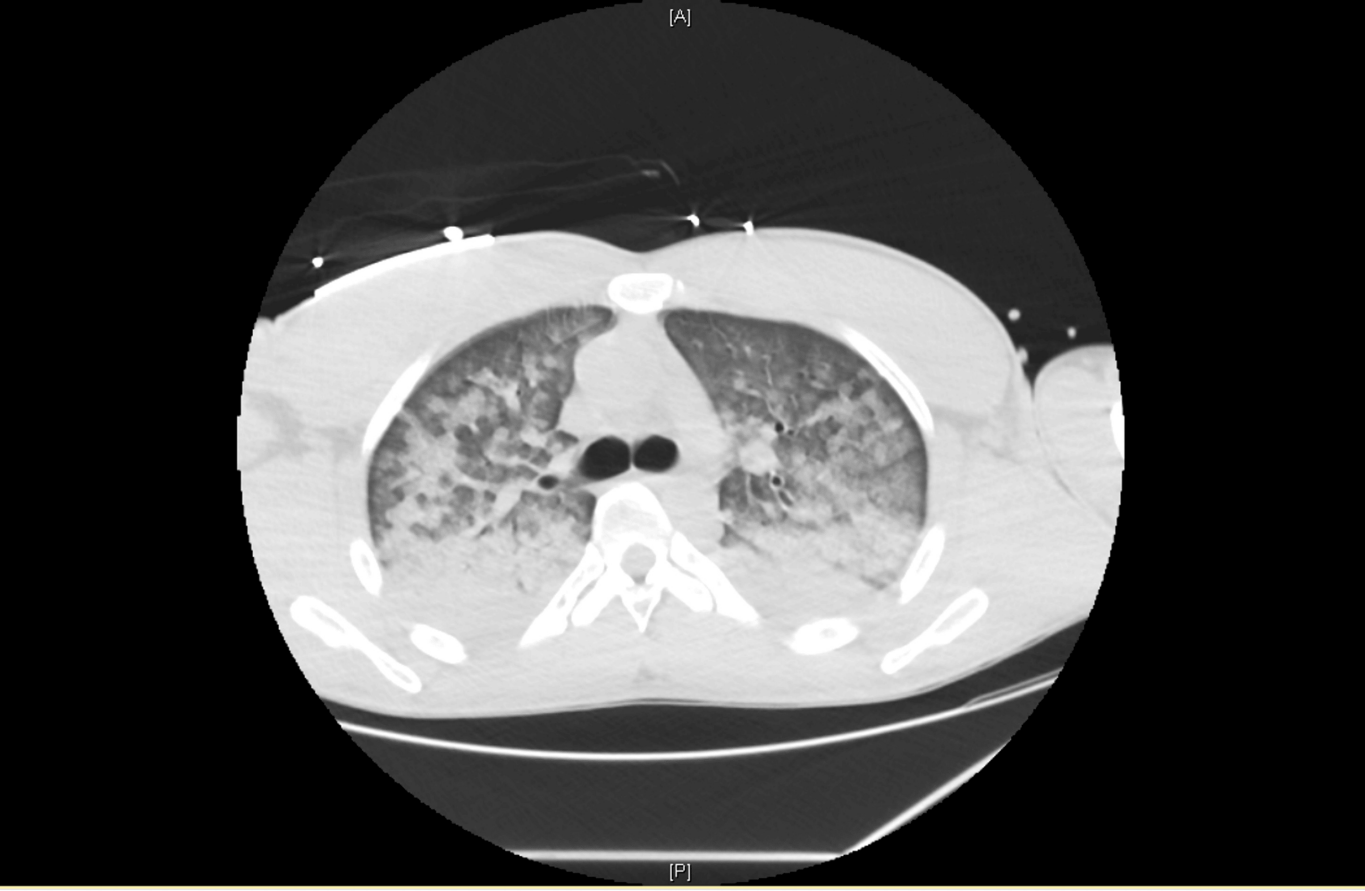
CT showing extensive bilateral pulmonary involvement with widespread ground-glass opacities.
